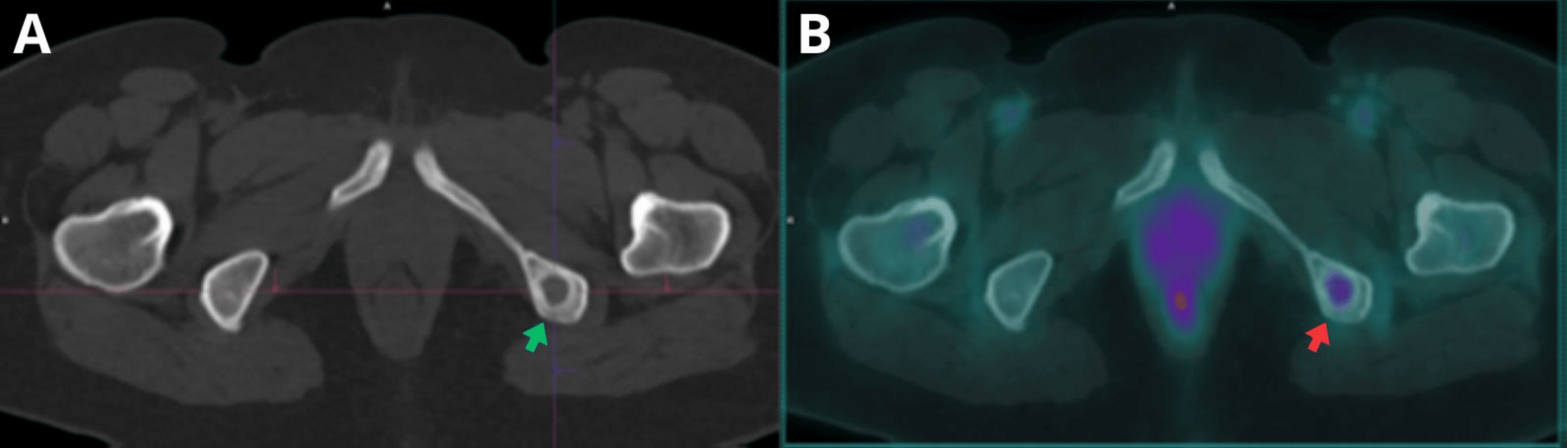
A) Axial CT scan showing an osteolytic lesion in the left ischium, measuring 1.4 cm (green arrow). B) Axial fused PET/CT image demonstrating hypermetabolism in the osteolytic lesion, SUVmax of 2.0 (red arrow). CT: computed tomography; PET/CT: positron emission tomography/computed tomography
